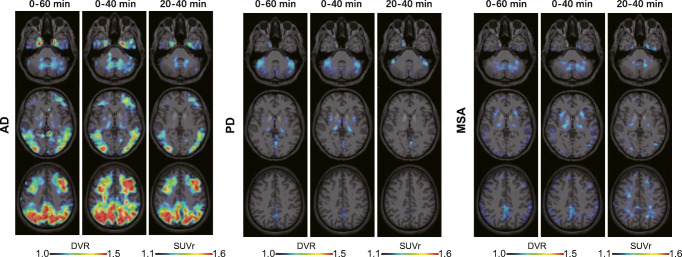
Representative [^18^F]PI-2620 images for 0–60 DVR, 0–40 DVR, and 20–40 SUVr in Alzheimer’s disease (AD) and α-synucleinopathies. Axial slices upon an MRI standard template show distribution volume ratios (DVR) and the standardized uptake value ratio (SUVr) of a patient with AD (female, 66 years, MMSE: 20), a patient with PD (female, 57 years, MoCA: 28, UPDRS: 25), and a patient with MSA-C (male, 55 years, MoCA: 26, UPDRS: 28)
